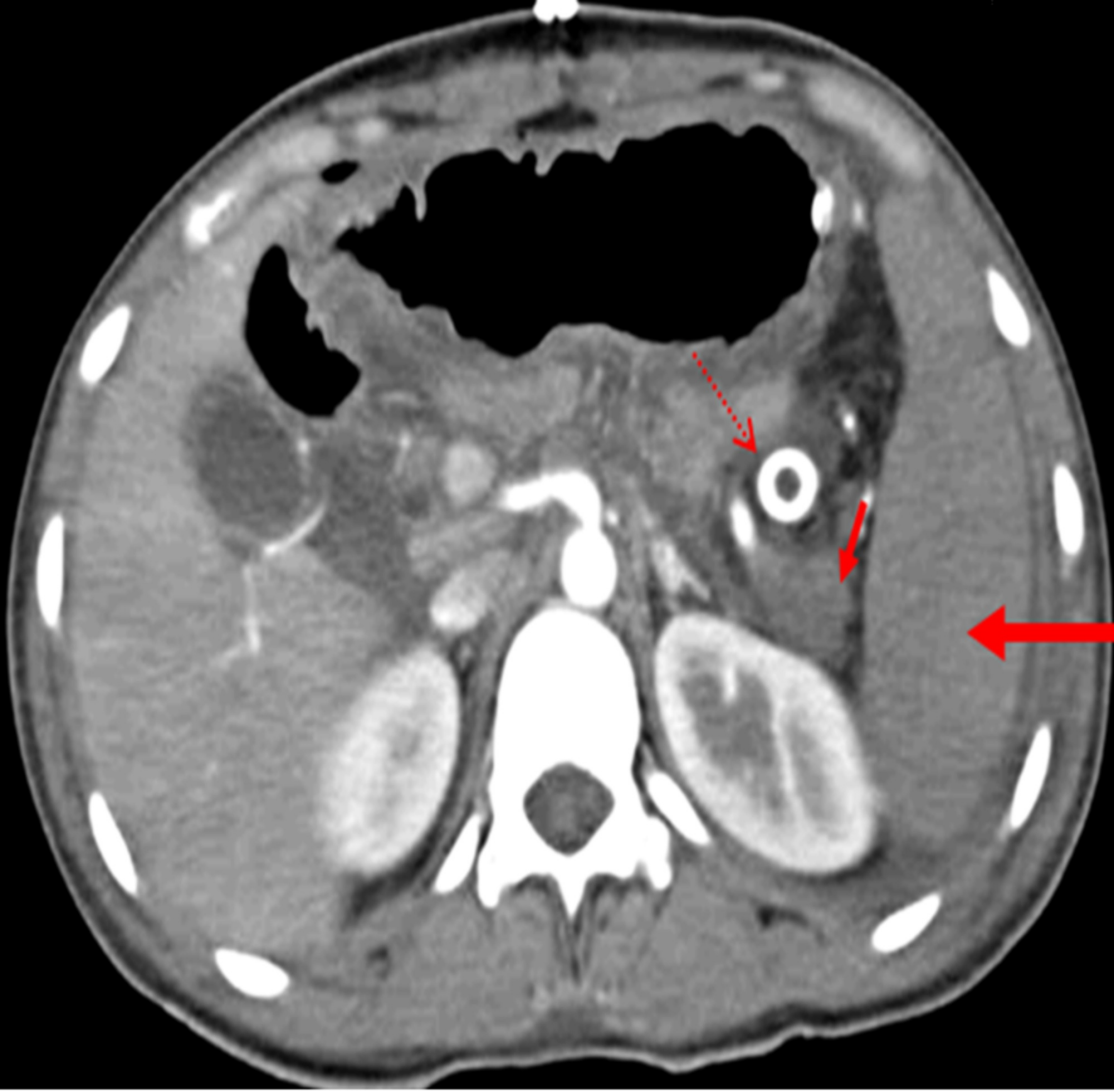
Post-operative contrast-enhanced computed tomography (CECT) angiography showing retrograde blood flow into the splenic artery within the distal pancreatic stump indicating distal pancreatic perfusion. Long arrow: enhancement of spleen; Short arrow: enhancement of distal pancreatic stump; Dotted arrow: abdominal drainage catheter.
